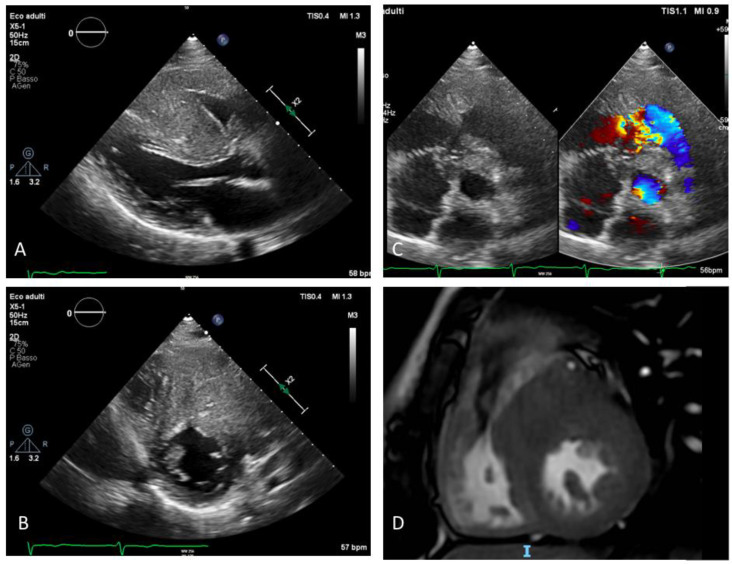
Preoperative imaging showing severe biventricular hypertrophy with right ventricular outflow tract obstruction: (A) Parasternal long axis view: severe hypertrophy of interventricular septum and of the RV free wall; (B) parasternal short axis view: severe hypertrophy of RV outflow tract and color Doppler aliasing due to restriction of outflow tract and consequent flow acceleration (C) parasternal short axis view: severe and asymmetric hypertrophy of IVS and RV free wall, (D) CMR focused on RV outflow tract: hypertrophy of the RV free wall and IVS. RV: right ventricle; IVS: interventricular septum; CMR: cardiac magnetic resonance.
